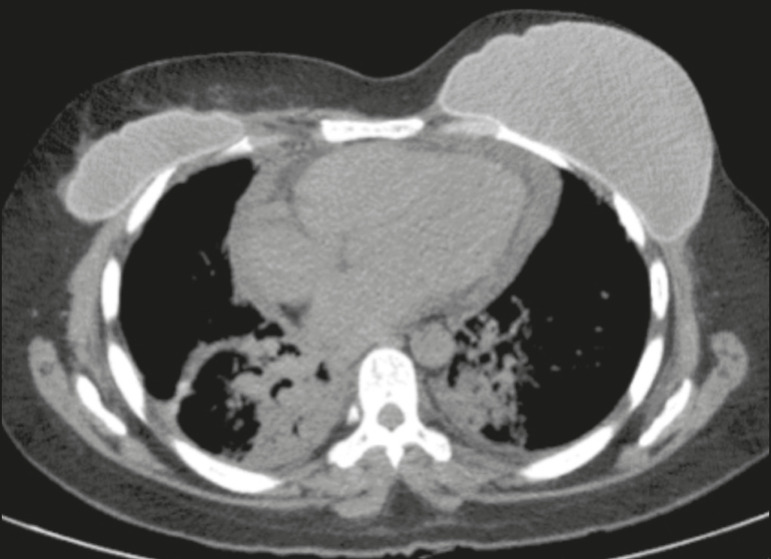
Unenhanced axial CT slice showing moderate pericardial effusion in a patient who had undergone radiotherapy of the left breast for the treatment of breast cancer.
